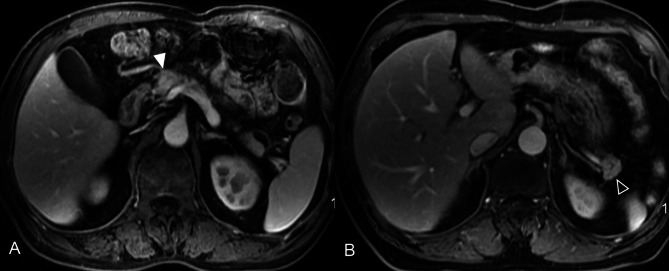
MRI of high-enhancement pancreatic ductal adenocarcinoma and mass-forming chronic pancreatitis. (A) Pancreatic ductal adenocarcinoma showed the higher enhancement than pancreatic parenchyma in portal venous phase (white narrow); (B) Mass-forming chronic pancreatitis showed higher enhancement than pancreatic parenchyma in portal venous phase (hollow narrow)
